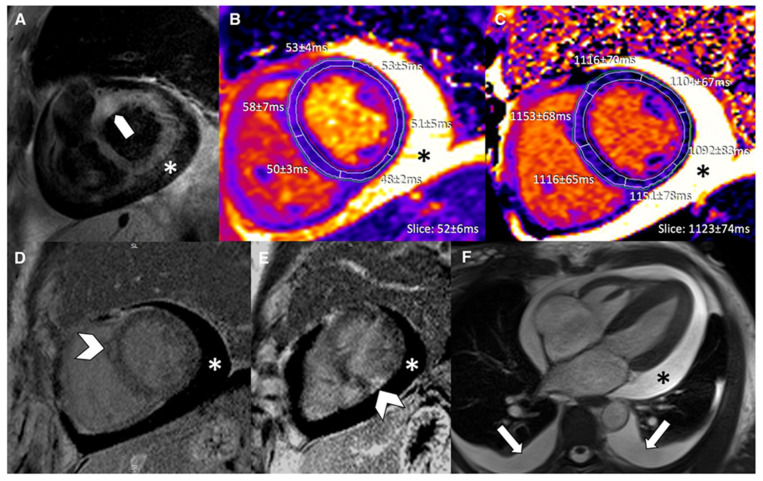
Multi-contrast cardiovascular magnetic resonance image panel in patient with immune checkpoint inhibitor myocarditis. (A) T2-weighted SPAIR, (B) T2 mapping, (C) native T1 mapping, (D,E) phase-sensitive inversion recovery late gadolinium enhancement imaging, and (F) cine steady-state free precession. Focal basal myocardial edema (block arrow), focal late gadolinium enhancement (arrowheads), moderate pericardial effusion (*), and mild bilateral pleural effusion (arrows) are demonstrated. Reproduced from: Wintersperger, B.J., et al. (2022) [58]. Licensed under CC BY-NC 4.0 (https://creativecommons.org/licenses/by-nc/4.0/ accessed on 15 December 2025).
